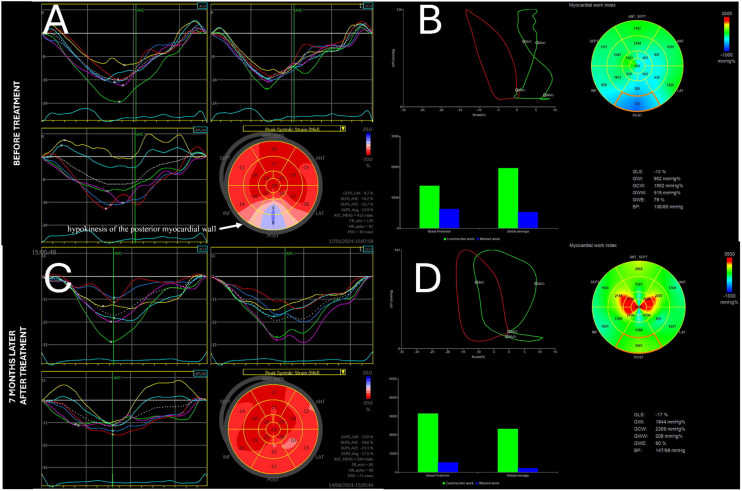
Echocardiographic evaluation of systolic function. (A)—GLS with hypokinesis of posterior myocardial wall. (B)—Myocardial work, dysfunction also visible in basal segment of posterior wall. (C)—GLS after treatment. Visible improvement. (D)—Improved myocardial work after treatment.
